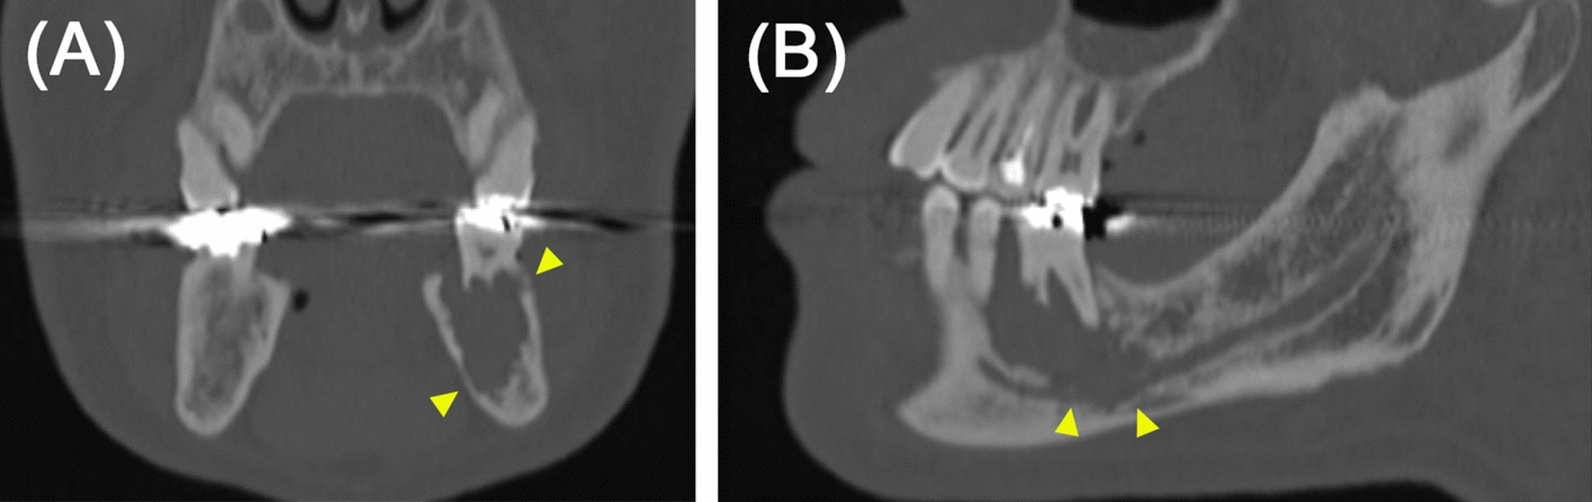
Imaging findings of the primary lesion. Coronal cone-beam computed tomography image shows a radiolucent lesion with an irregular border and no expansion of the cortical bone (A, arrowhead). Sagittal cone-beam computed tomography image shows the disappearance of the mandibular canal wall (B, arrowhead)
